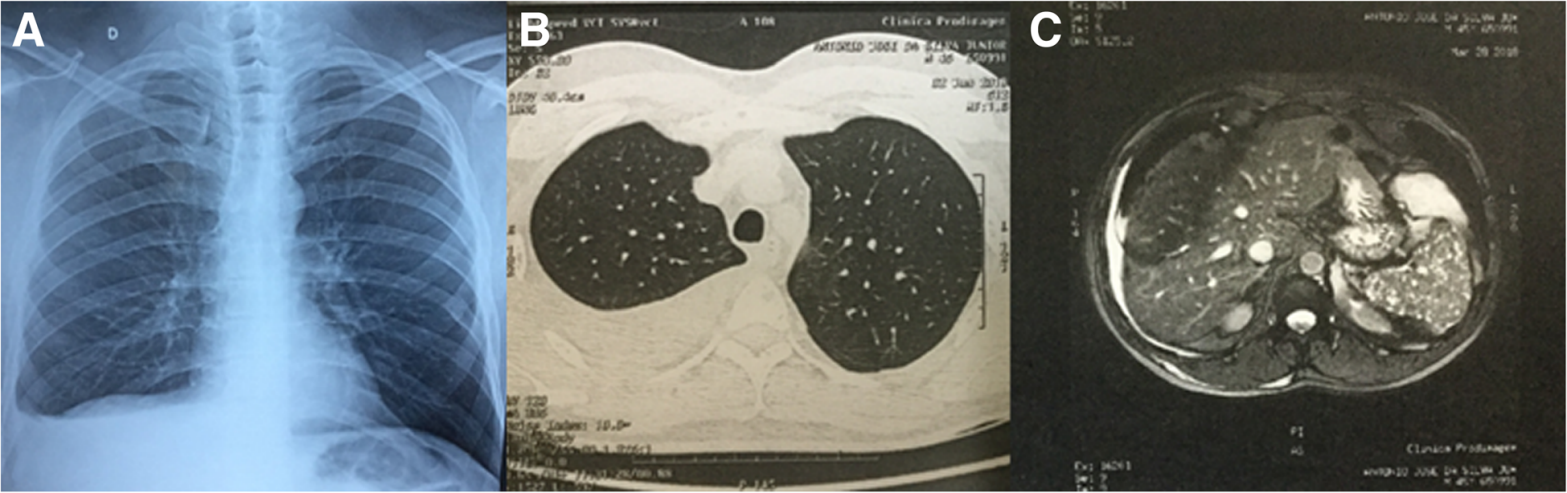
a Posteroanterior (PA) chest x-ray showing pleural effusion on the right. b Chest computed tomography with contrast enhancement showing pleural effusion in the right hemithorax. c Magnetic resonance imaging (MRI) showing multiple lytic lesions in the spleen parenchyma
